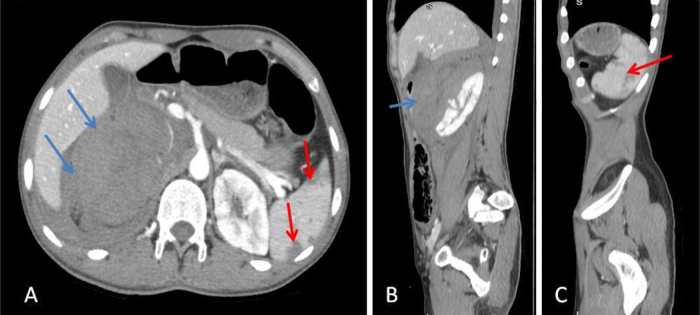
Axial (A), right parasagittal (B), and left parasagittal (C) views of post-contrast-enhanced computed tomography scan show retroperitoneal hematoma with contrast extravasations (blue arrows) and multiple splenic infarctions (red arrows).
